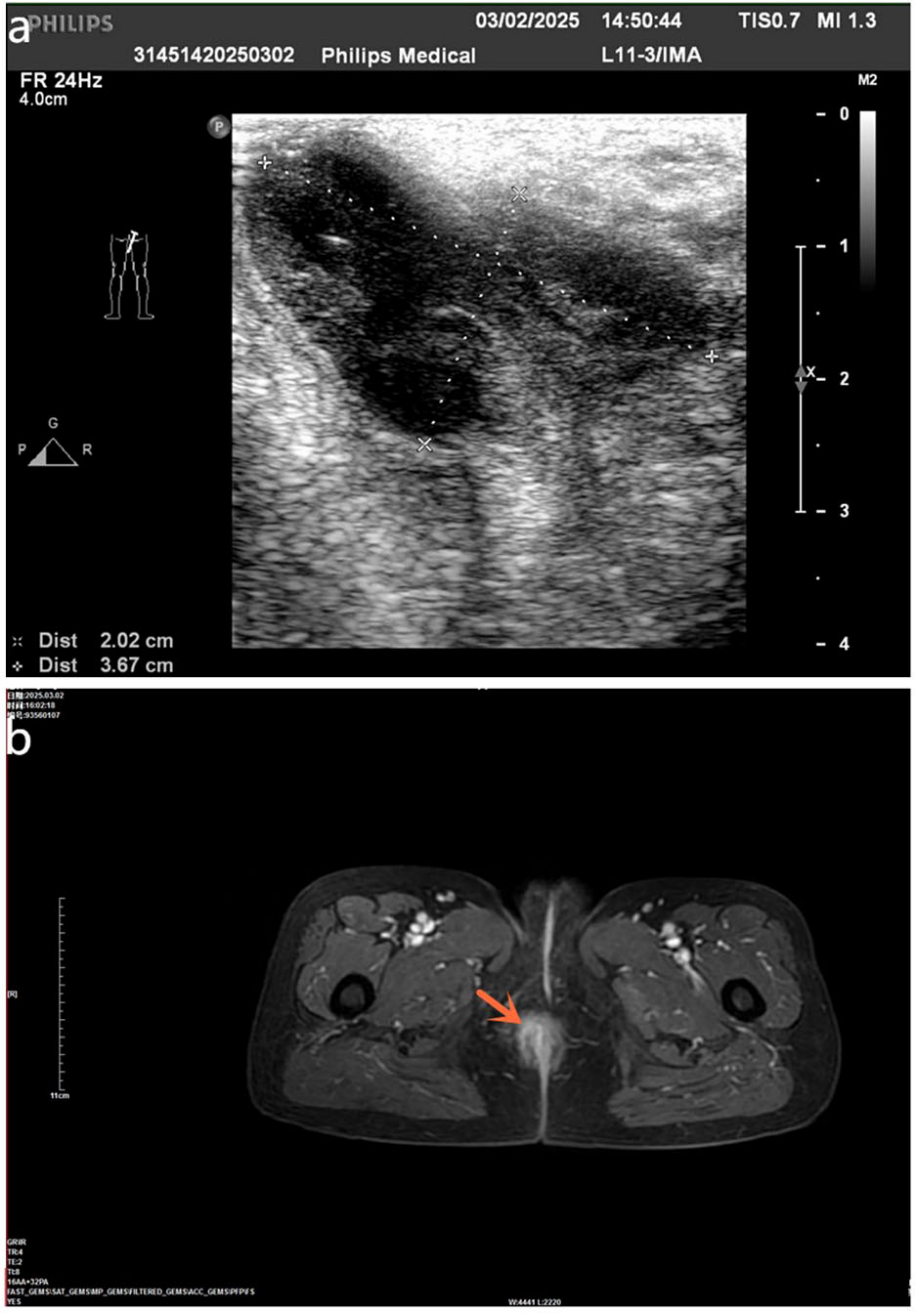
(a) Preoperative B-ultrasound examination showed a low heterogeneous echo area (dashed line between two “+” and dashed line between two “×”), with visible boundary and irregular shape in the right perianal area, adjacent to the subcutaneous area. (b) Pelvic MRI enhancement showed a thickened right lateral wall of the anal canal, which was isointense on T1-weighted imaging (T1WI), as well as hyperintense on T2WI and hyperintense on DWI, with obvious ring enhancement after contrast (red arrows).
